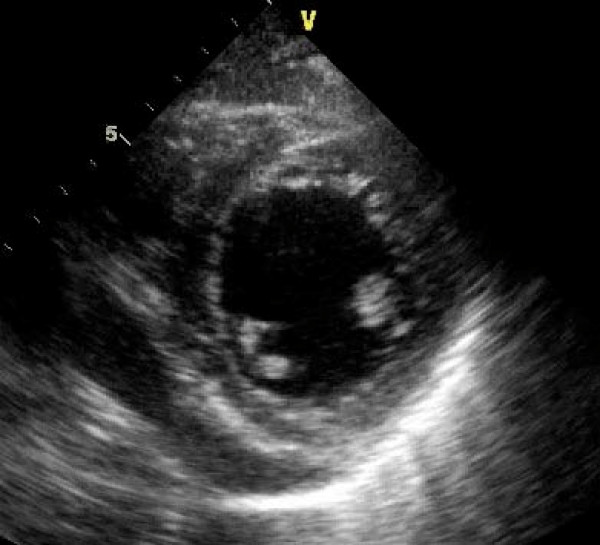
A transthoracic echocardiogram parasternal short axis view demonstrating a mildly dilated left ventricle and global LV systolic dysfunction.
